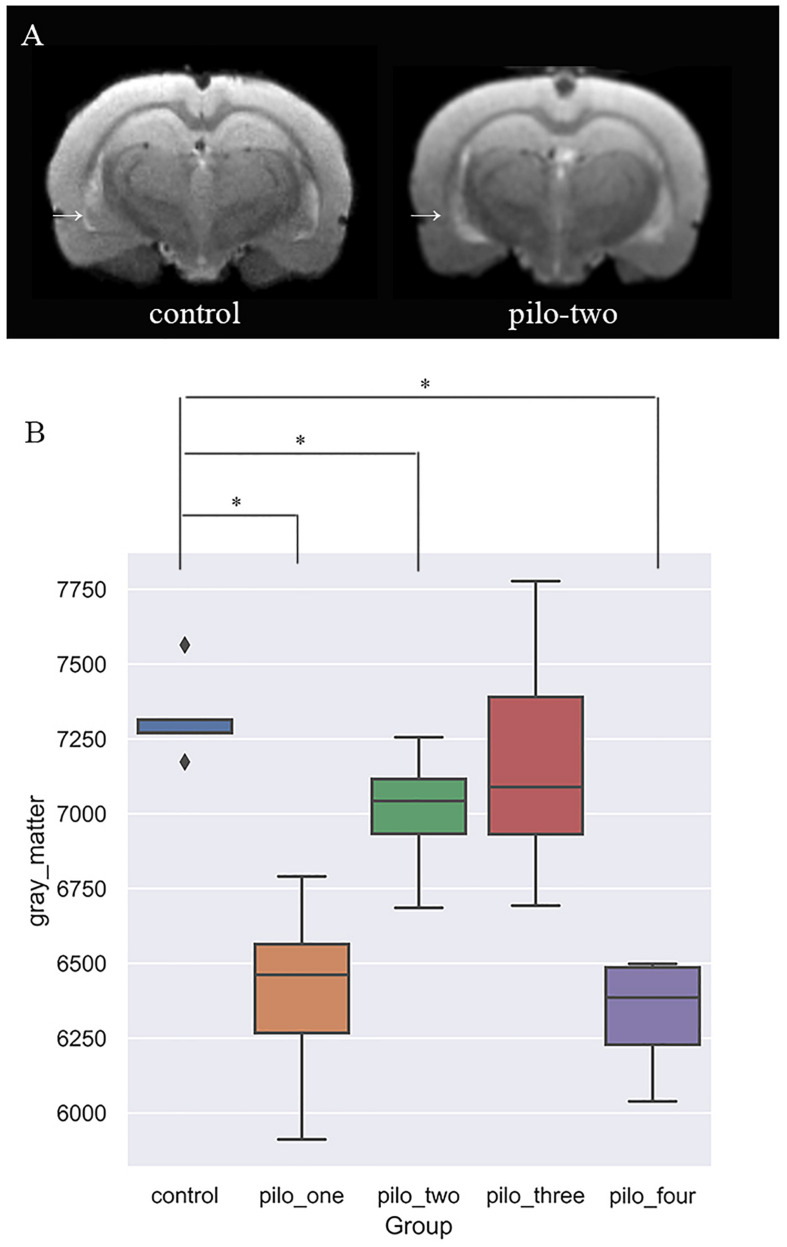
(A) T2-weighted image showing larger ventricular volumes after status epilepticus (SE). *Arrow*: larger lateral ventricular volume than the control. (B) Gray matter volumes decreased after SE, especially 1 month after SE (P* < 0.05).
